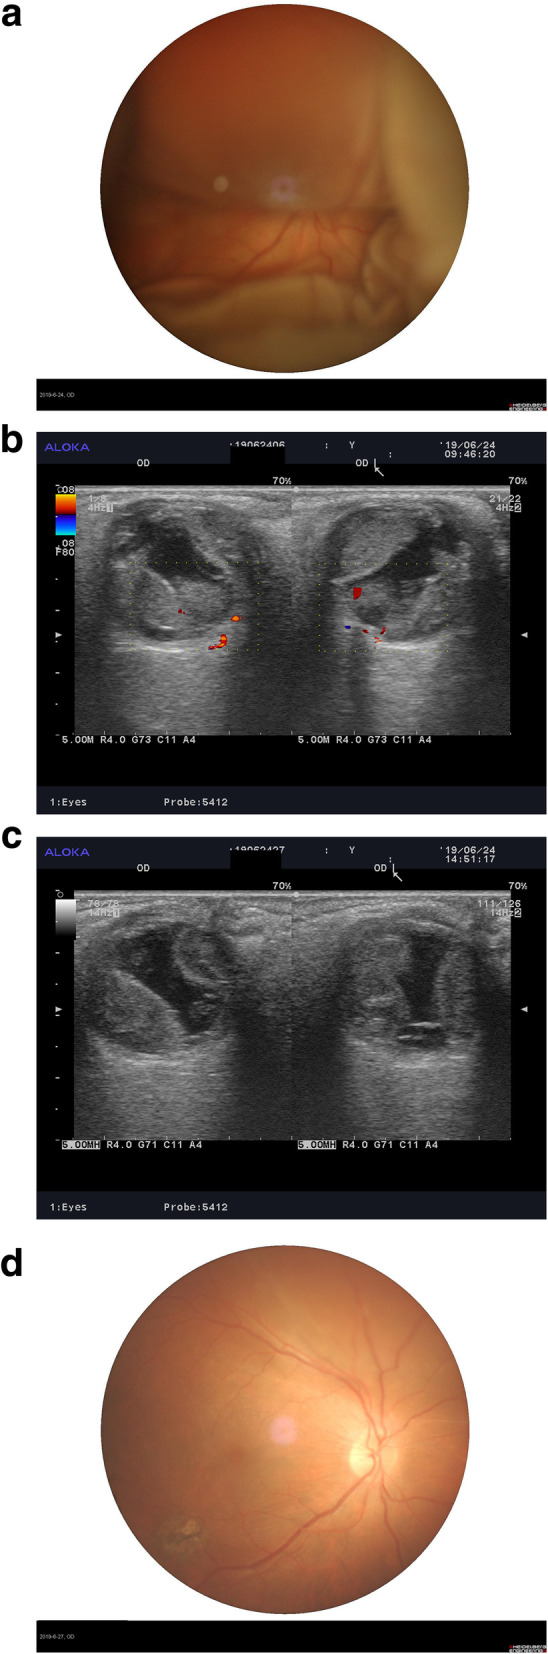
Fundus image and ultrasound examination of Case 2. a is a fundus image of the fourth day of DSCH; b is an ultrasound examination of the fourth day before rt-PA injection; c is an ultrasound examination showed partial liquidation of SCH 4 h after rt-PA injection; d is a fundus image of the third day after choroidal drainage showing that a bulge can only be seen in the upper choroid
